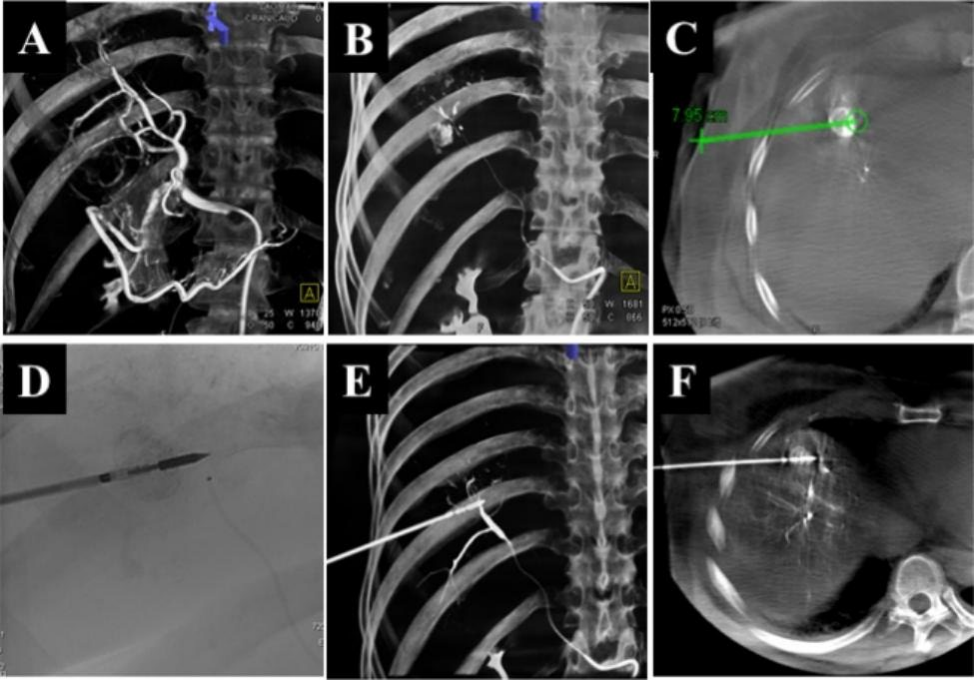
Patients with HCC with a diameter of 3 mm adjacent to the diaphragm; A. The tumor first completes the transcatheter arterial chemoembolization under the guidance of CBCT;B. HCC is marked by iodized oil; C. preoperative puncture route planning under the guidance of CBCT; D-F. The patient completes the MWA process immediately after TACE treatment
